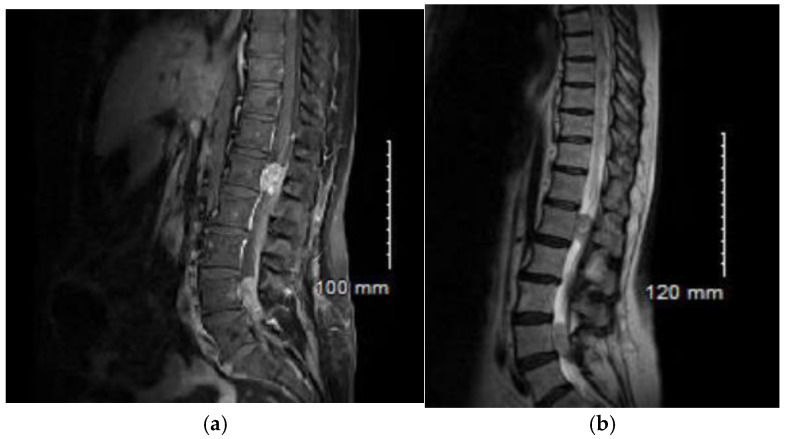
Sagittal magnetic resonance imaging (MRI) showing leptomeningeal carcinomatosis: (a) T1-weighted sequence after gadolinium administration; (b) T2-weighted FLAIR sequence.
